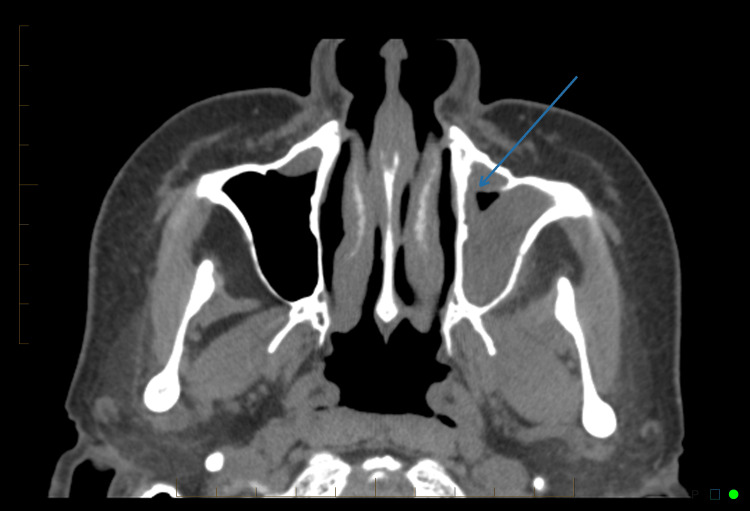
Axial non-contrast CT of the paranasal sinuses demonstrating asymmetric mucosal thickening of the left maxillary sinus (blue arrow), more marked than the contralateral side, without evidence of bony destruction CT: computed tomography
